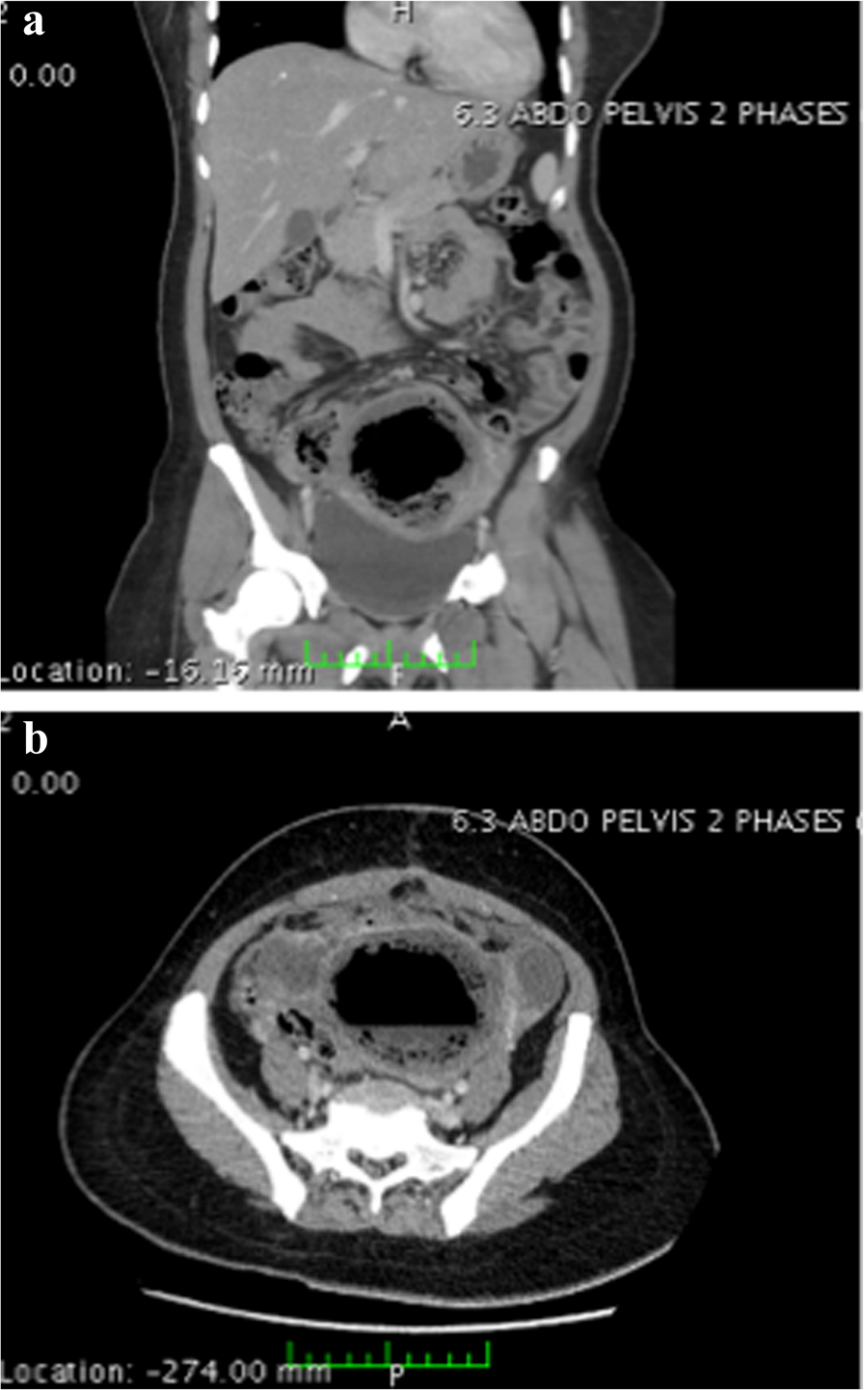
Abdominal computed tomography scan revealing hydroaeric levels inside the uterus. a: coronal view. b: transverse view.
